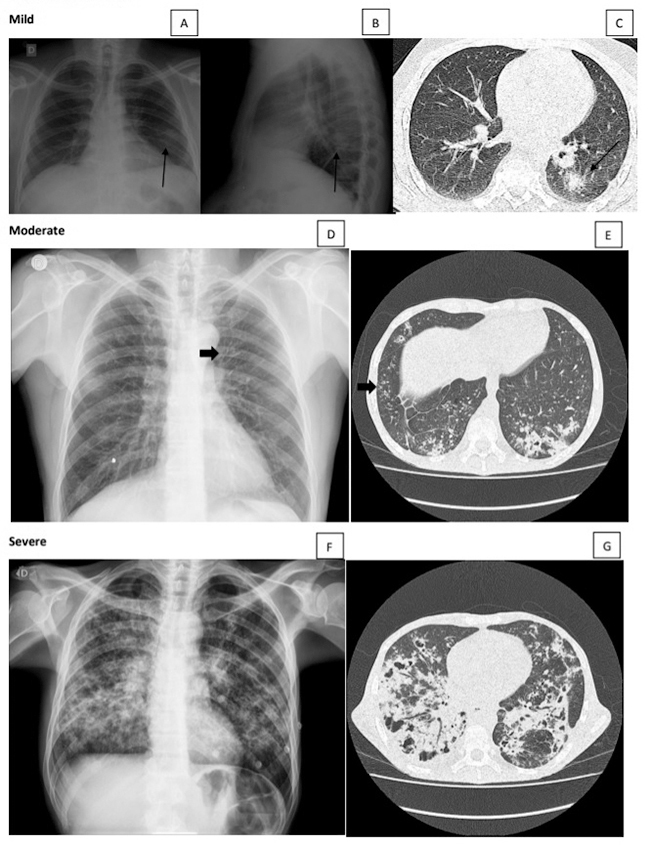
(A, B, C) Mild active lesion. Chest radiograph and computed tomography (CT) scan of the same patient showing a solitary pulmonary nodule. Moderate active lesion: (D) chest radiograph showing intersticial lesions with bronchial wall thickening (arrow) and (E) CT scan showing consolidation, ground-glass opacities, and intersticial lesions with tree-in-bud pattern (arrow). (F, G) Severe active lesion. Mixed lesions involving > 1/3 of the total pulmonary parenchyma with cavitary lesions.
